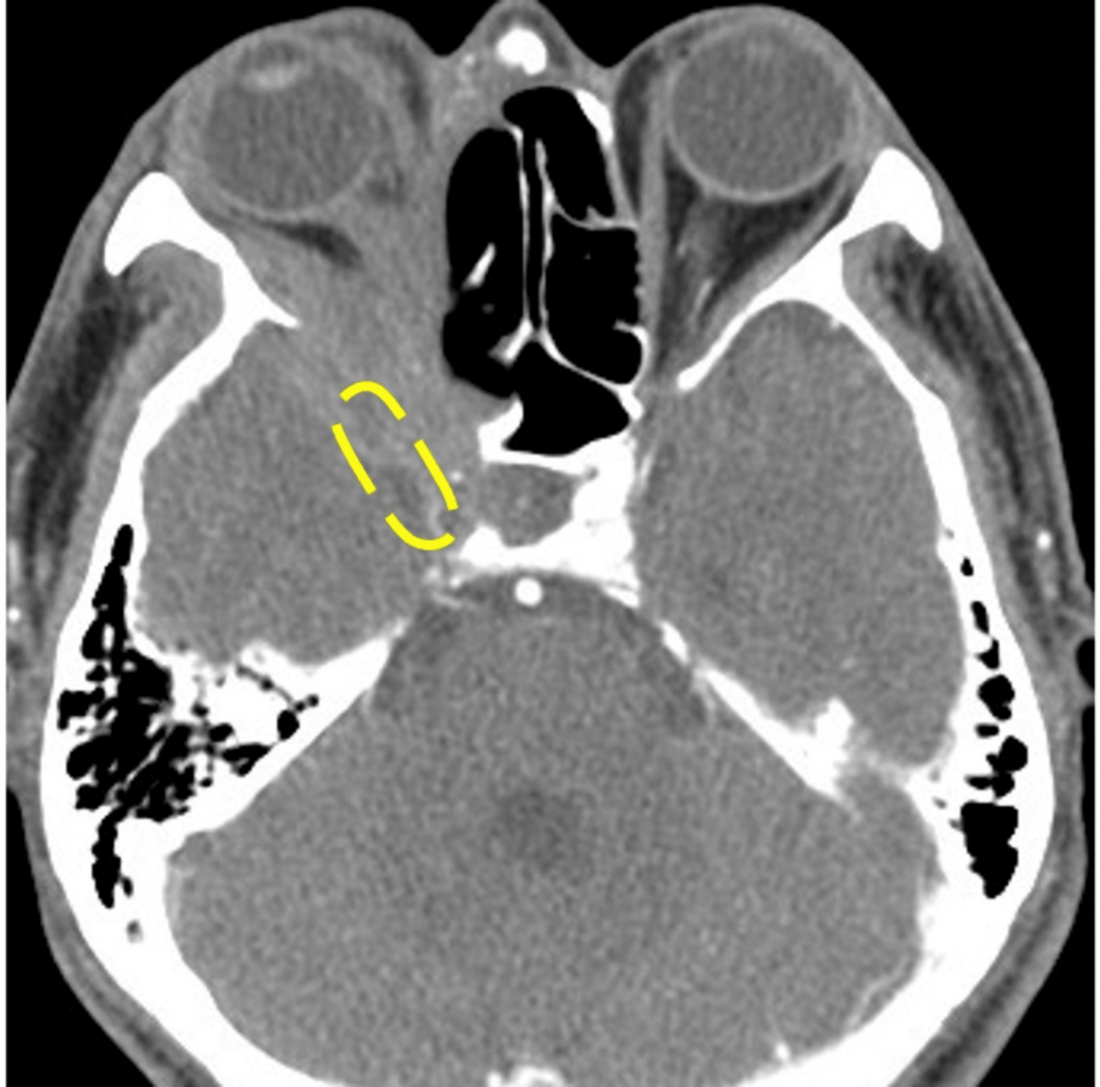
Contrast-enhanced CT of the sinus eight months after surgery (axial section) The lesion at the right orbital apex had shrunk (dotted line).
